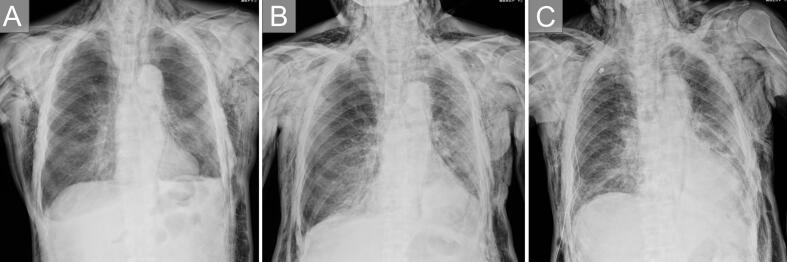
(A) Subcutaneous and mediastinal emphysema seen on the chest X-ray at readmission. A slight suspicion of right pneumothorax. (B) Chest X-ray 4 h later showing progression of the right pneumothorax. (C) Right lung expansion is observed after chest drainage.
